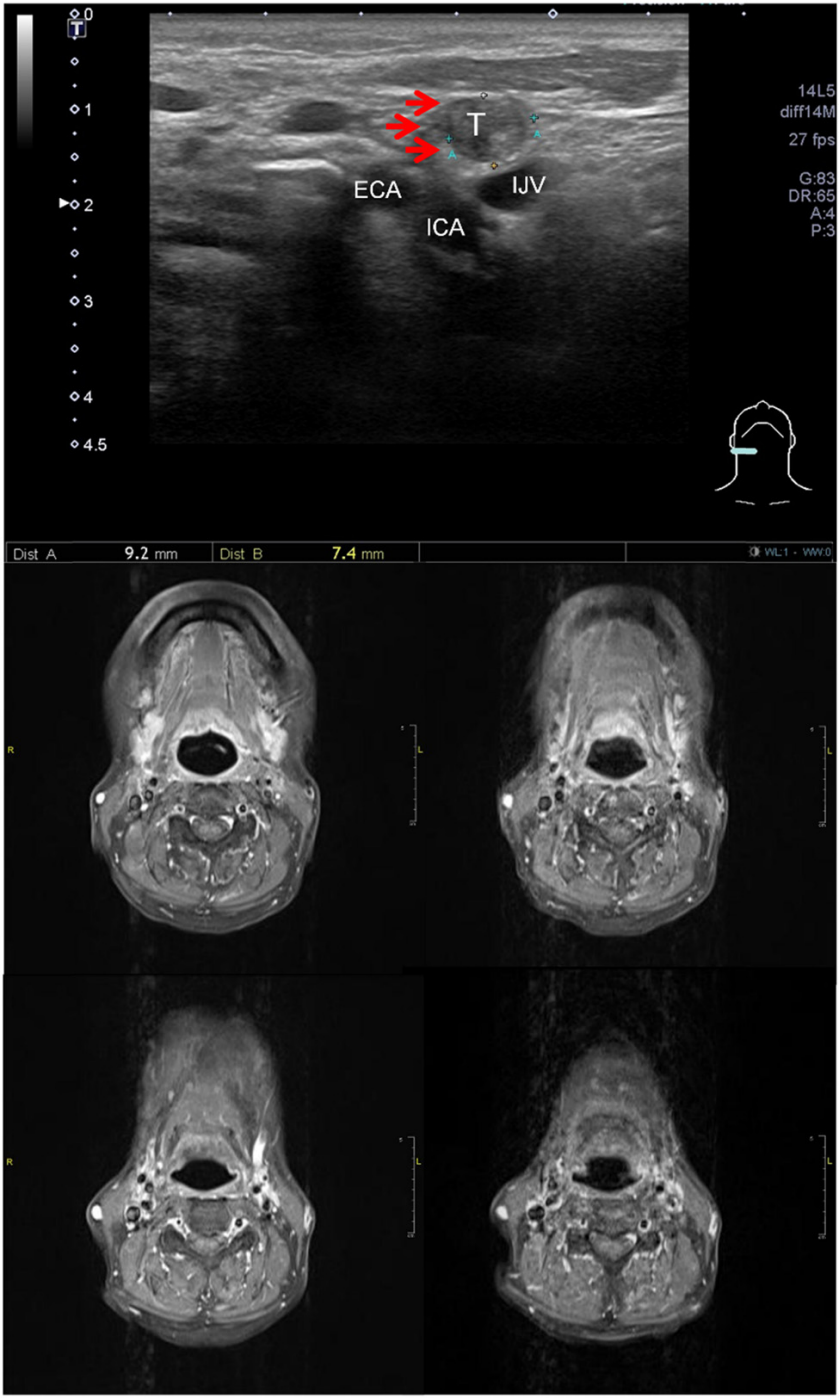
One representative patient. A 59-year-old male was diagnosed with oropharyngeal cancer, stage IVA and received definite CCRT. The follow-up MRI showed no abnormal cervical LN. US showed a round, ill-defined, heterogeneous, and hypoechogenic mass (0.74 cm × 0.92 cm) at right level II (arrow). The cytological and further pathological reports both showed metastatic squamous cell carcinoma. Abbreviations: T, tumor; ECA, external carotid artery; ICA, internal carotid artery; IJV, internal jugular vein.
